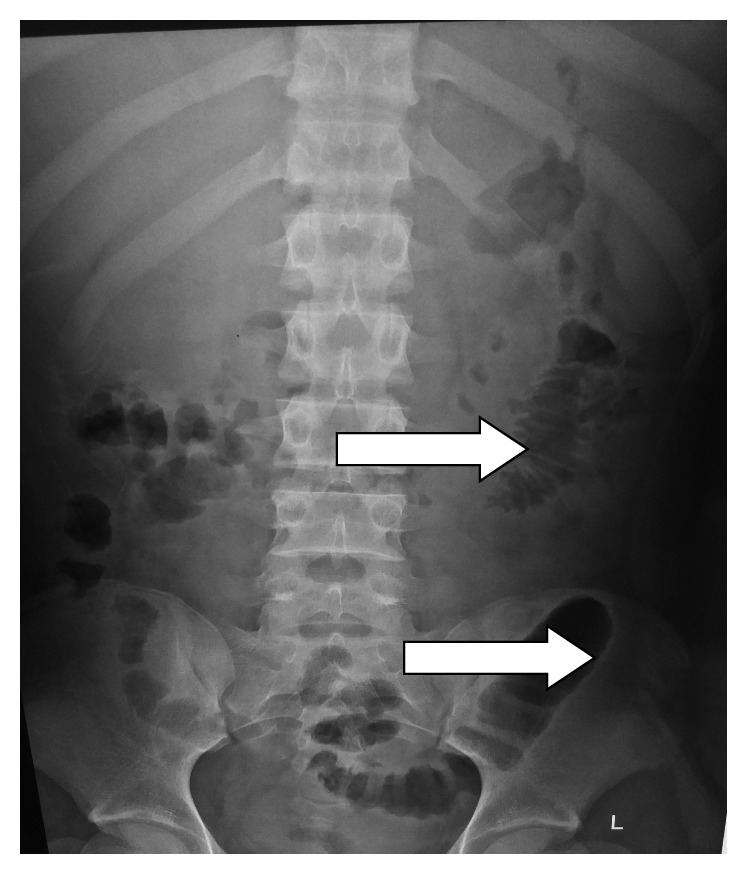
Sentinel loop sign and colon cut-off sign in a similar plain abdominal radiograph.
